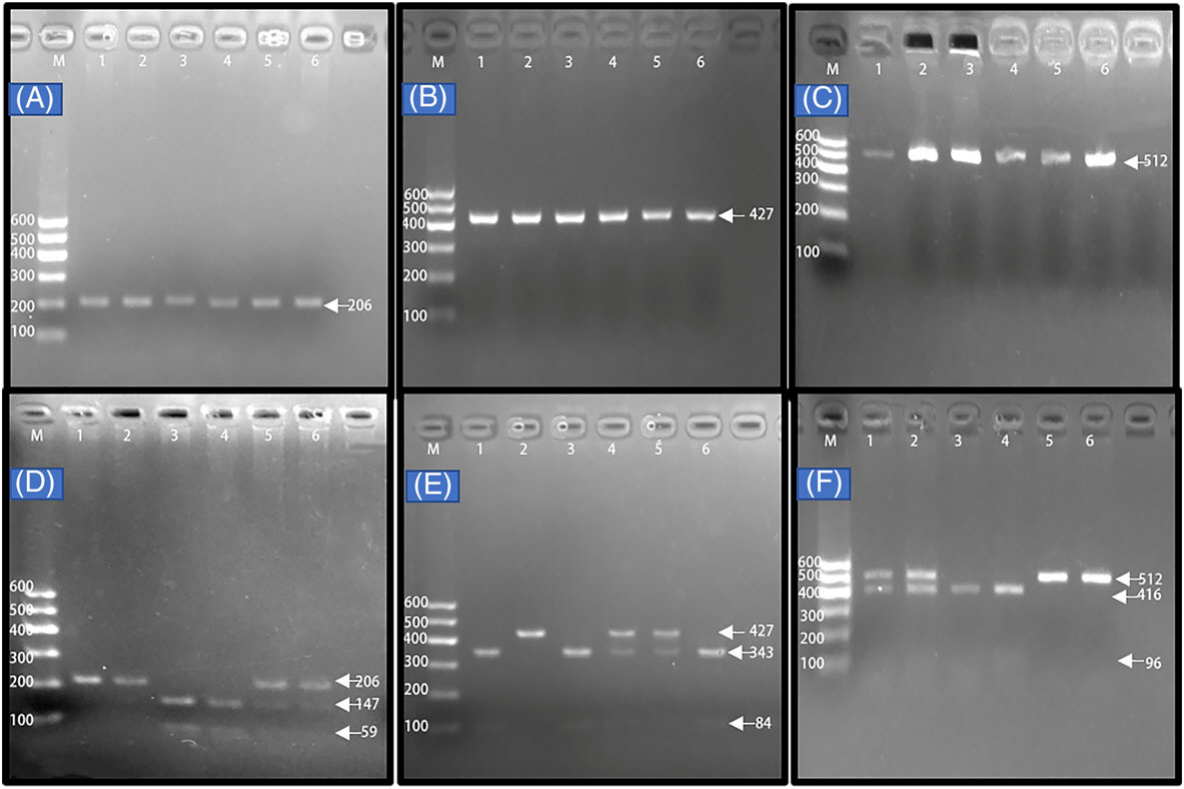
The PCR products of the samples and the genotypes of the *LIPG* SNPs. Lane M is the 100‐bp marker ladder; (A to C) lanes 1–6 are the PCR products: A, rs2156552 SNP (206 bp); B, rs4939883 SNP (427 bp); C, rs7241918 SNP (512 bp); D, genotyping of the rs2156552 SNP: lanes 1 and 2, TT genotype (206 bp); lanes 3 and 4, AA genotype (147 and 59 bp); and lanes 5 and 6, AT genotype (206, 147 and 59 bp); E, genotyping of the rs4939883 SNP: lanes 1, 3 and 6, CC genotype (343 and 84 bp); lanes 2, TT genotype (427 bp); and lanes 4 and 5, CT genotype (427, 343 and 84 bp); F, genotyping of the rs7241918 SNP: lanes 1 and 2, GT genotype (512‐, 416‐ and 96‐bp); lanes 3 and 4, GG genotype (416 and 96 bp); and lanes 5 and 6, TT genotype (512 bp)
